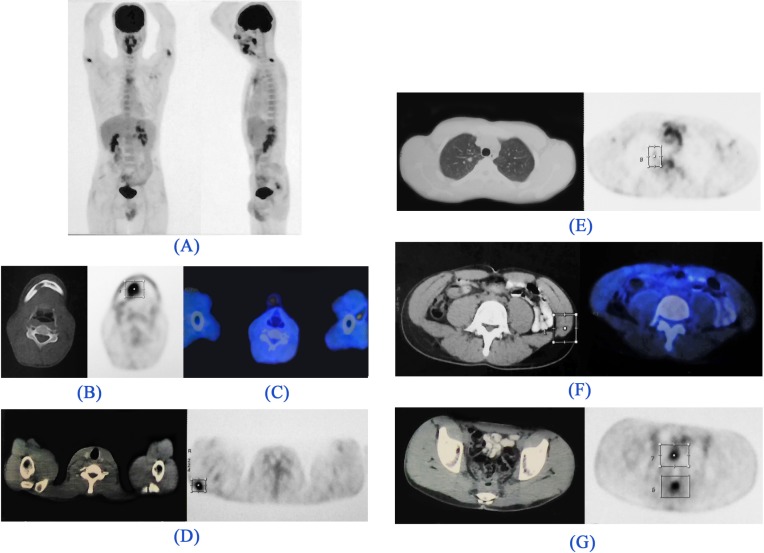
^18^FDG-PET/CT images. *(A). Maximum intensity projection (MIP) image:* There are foci of metabolically active lesions in the right paramedian submandibular region, bilateral proximal upper extremities, and posterior pelvic cavity. Metabolically active lesions are demonstrated in the *(B). Trans-axial CT (left) and PET (right) images:* Right submandibular region (right external lingual muscles) with invasion to the adjacent mandible, *(C). Trans-axial Fused image:* Posterior to the left biceps muscle, *(D). Trans-axial CT (left) and PET (right) images:* Right deltoid muscle, *(E).*
